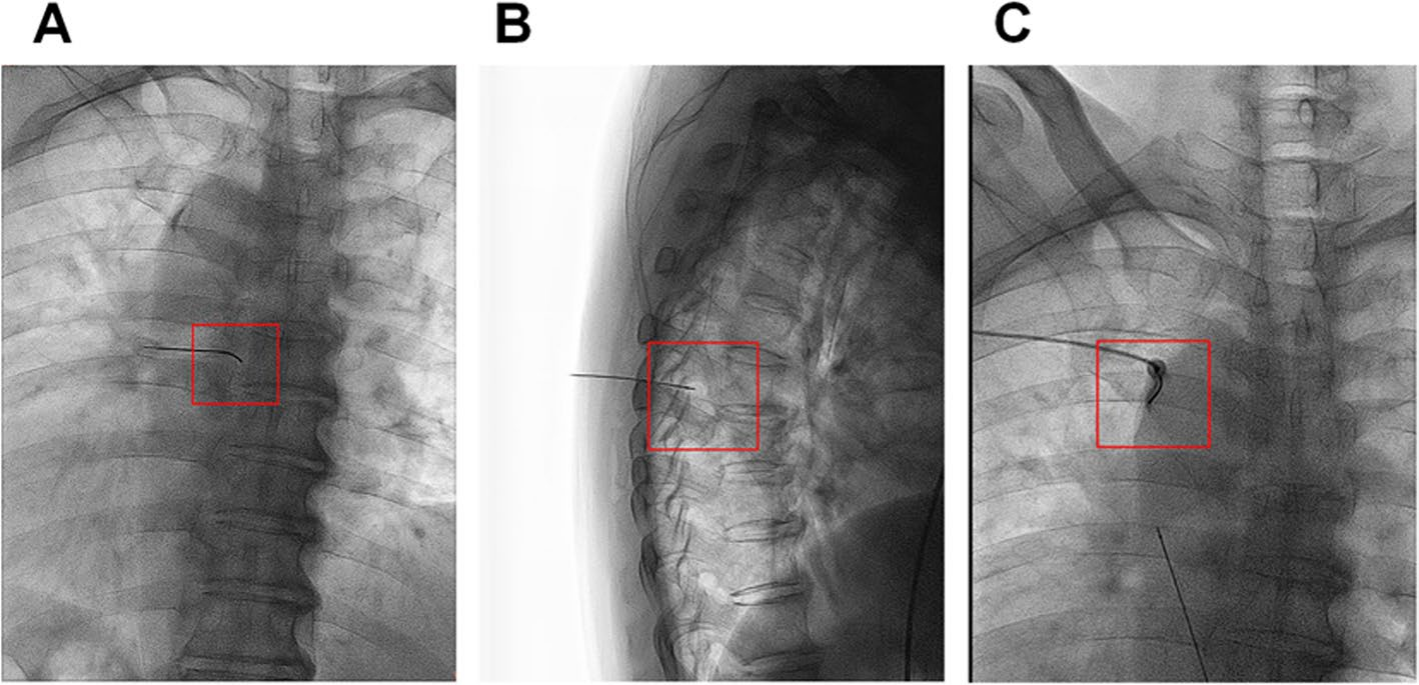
X-ray photo of PRF treatment. A X-ray imaging of PRF treatment on DRG in the anteroposterior view. B X-ray imaging of PRF treatment on DRG in the lateral view. C X-ray imaging of PRF treatment on ICN in the anteroposterior view. PRF, pulsed radiofrequency. DRG, dorsal root ganglion. ICN, intercostal nerve radiofrequency
